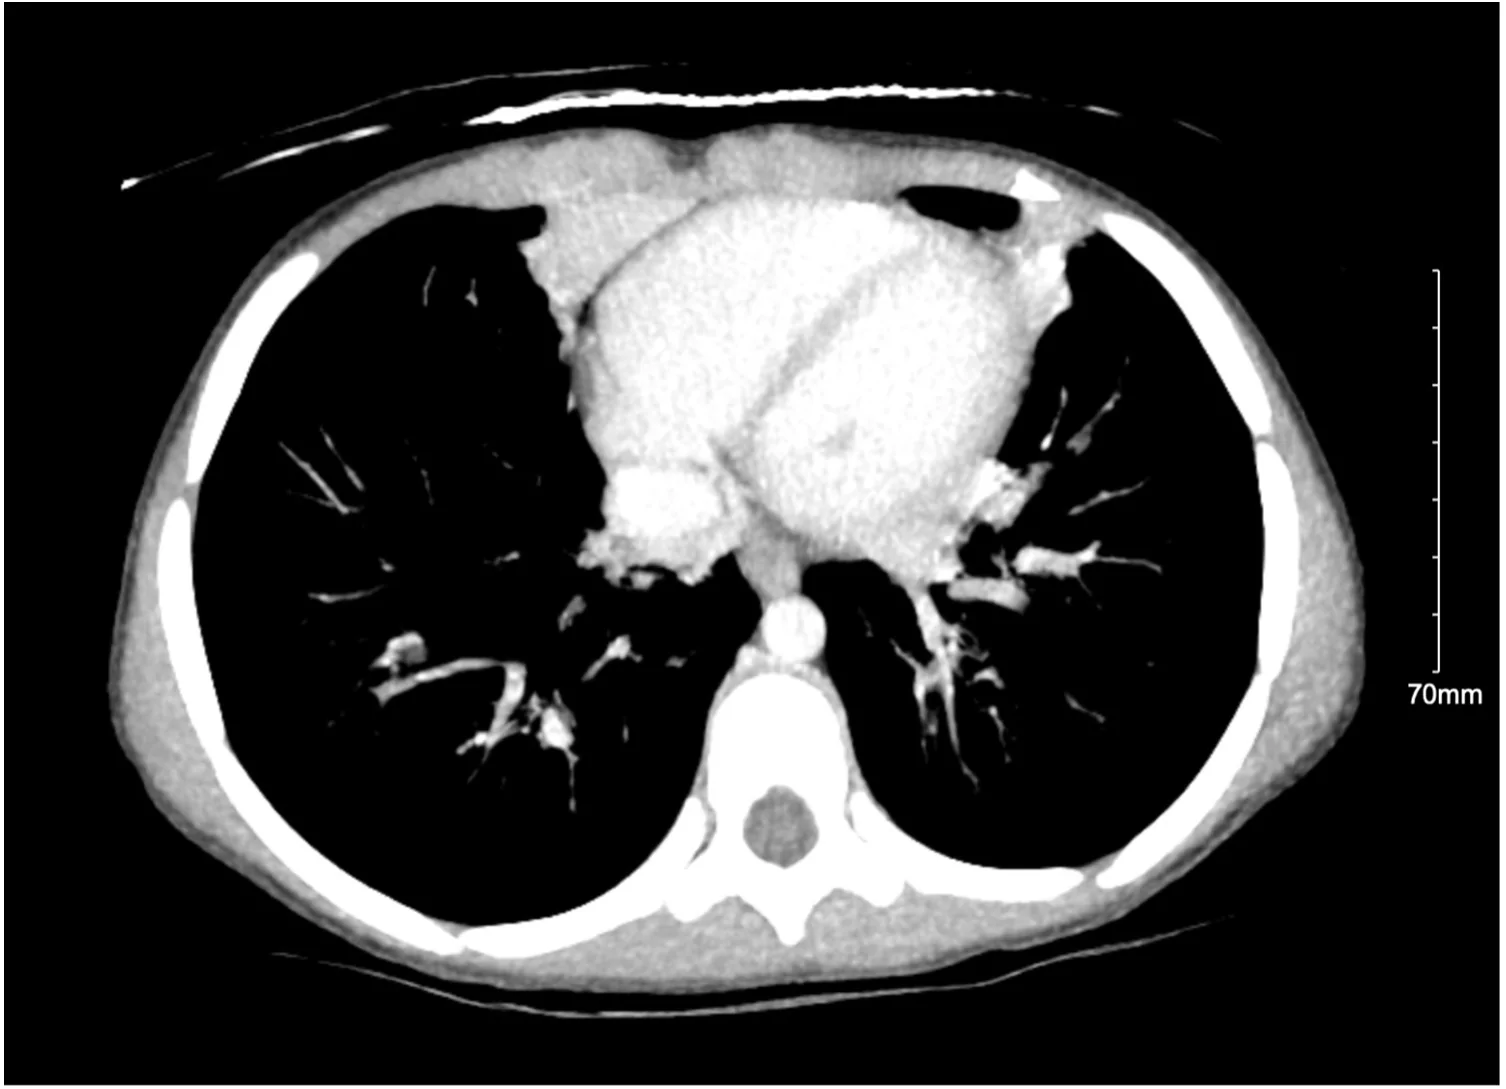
Axial contrast-enhanced CT of the chest at the level of the lower lobes demonstrating diffuse bronchial wall thickening with peribronchial cuffing bilaterally, mosaic attenuation consistent with air trapping, and subcarinal lymphadenopathy. No focal consolidation, pleural effusion, or central bronchiectasis is identified. These findings are consistent with a diffuse small airway inflammatory process in the context of prolonged earthquake rubble dust inhalation.
